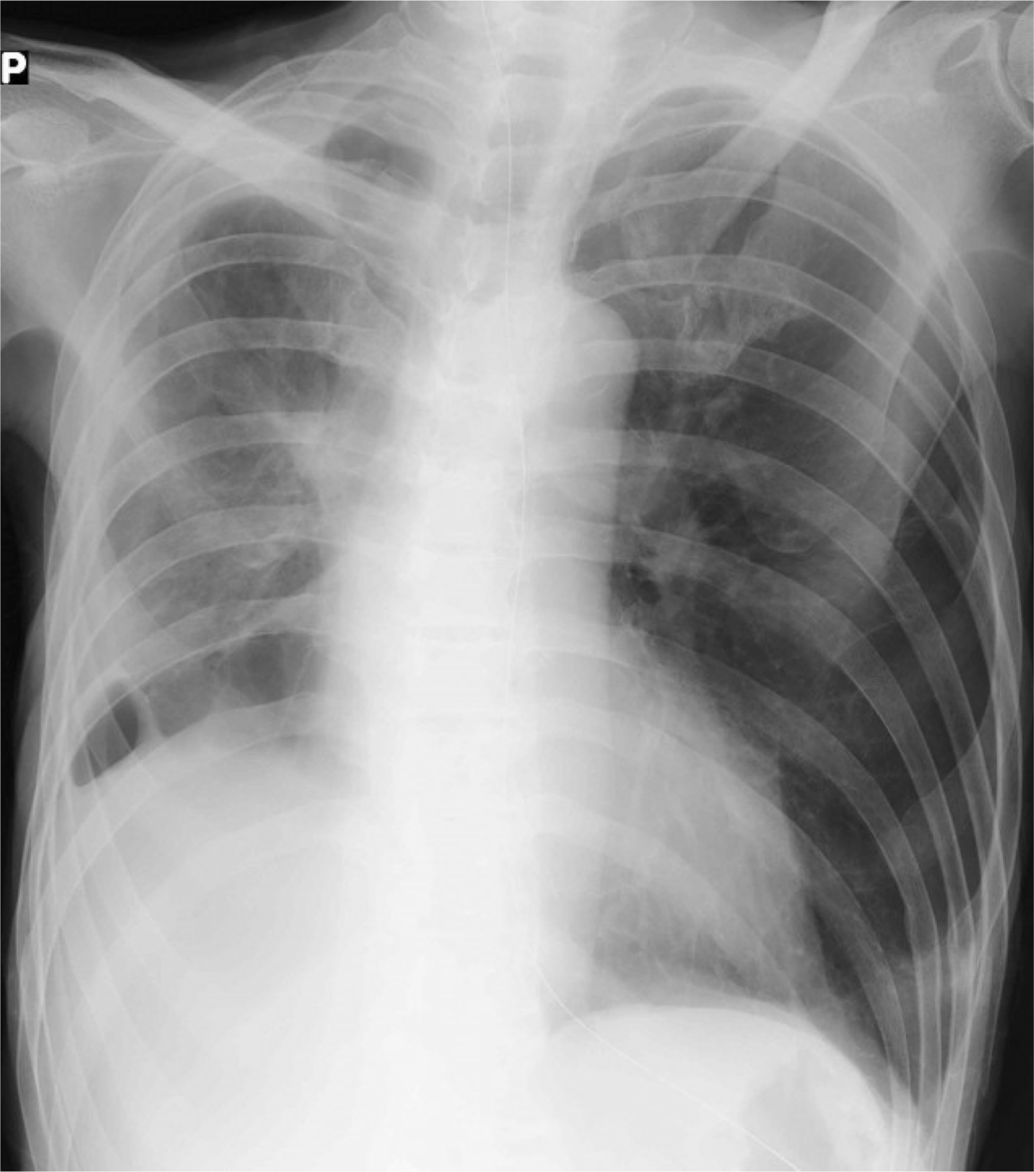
Chest radiograph shows that surgical intervention led to improvement in the infection.
